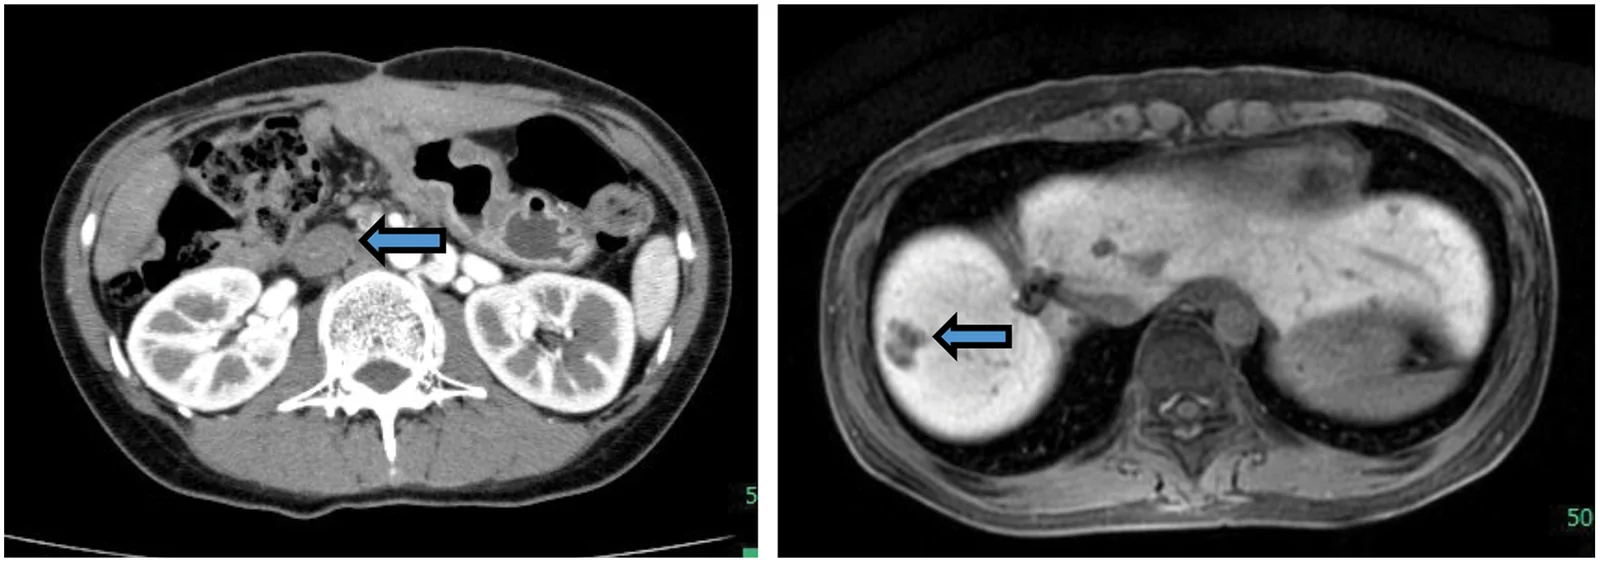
CT showing para-aortic lymphadenopathy and MRI revealing hepatic metastases.
